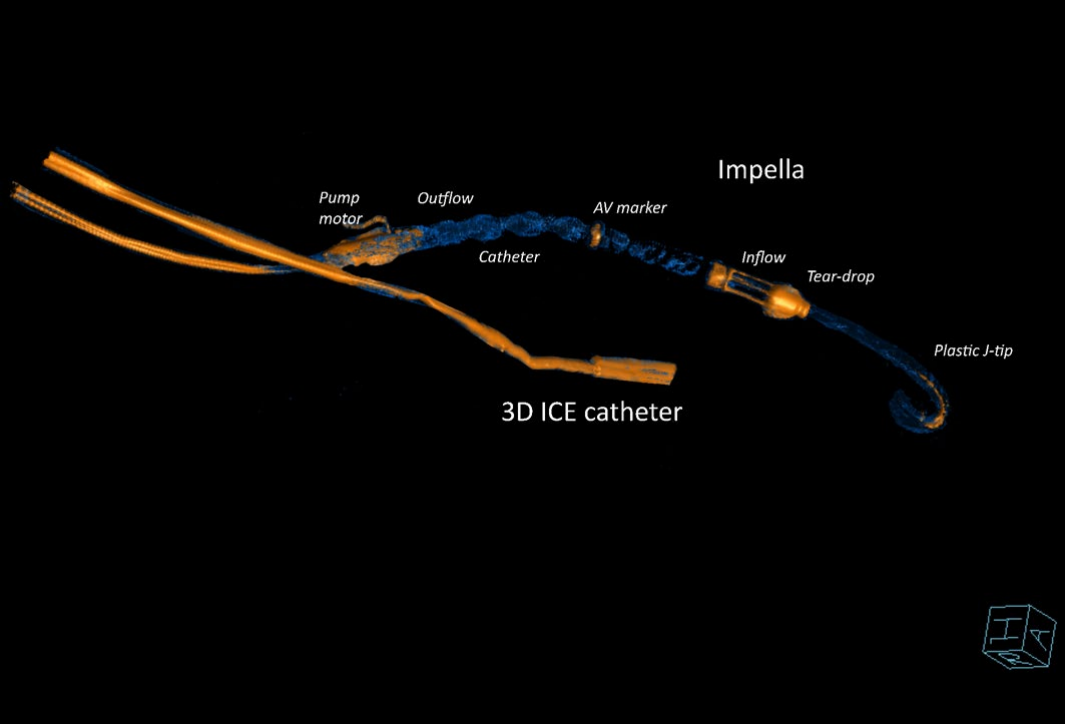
Fluoroscopy with 3D reconstruction has been performed to demonstrate favourable for imaging relationship between the Impella CP implanted into left ventricle and correctly positioned and the 3D ICE catheter with the ultrasound tip located within the right atrium. The proximity to the implanted device allows the use of high ultrasound scanning frequencies, contributing to improved axial spatial resolution. The 2D scanning plane is at a significant angle to the implanted Impella plane, thus requiring significant physical probe manipulations. 3D wide-angle acquisition rectifies this problem by acquiring full volume pyramid of data with elevation of 50° which was sufficient for adequate imaging of the Impella with minimal physical manipulations of the probe when using jugular venous access.
